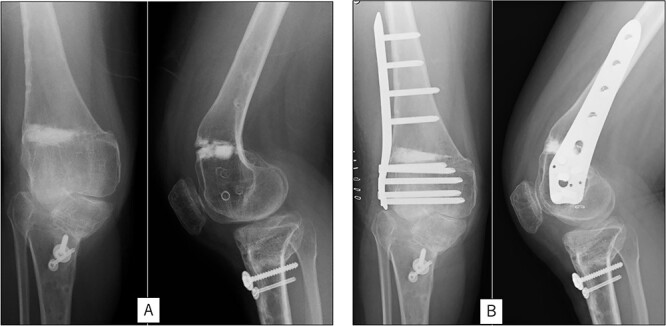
Radiography after fracture at the osteotomy of the right femur. (A) anteroposterior and lateral view immediately after fracture. (B) After open reduction and internal fixation.
